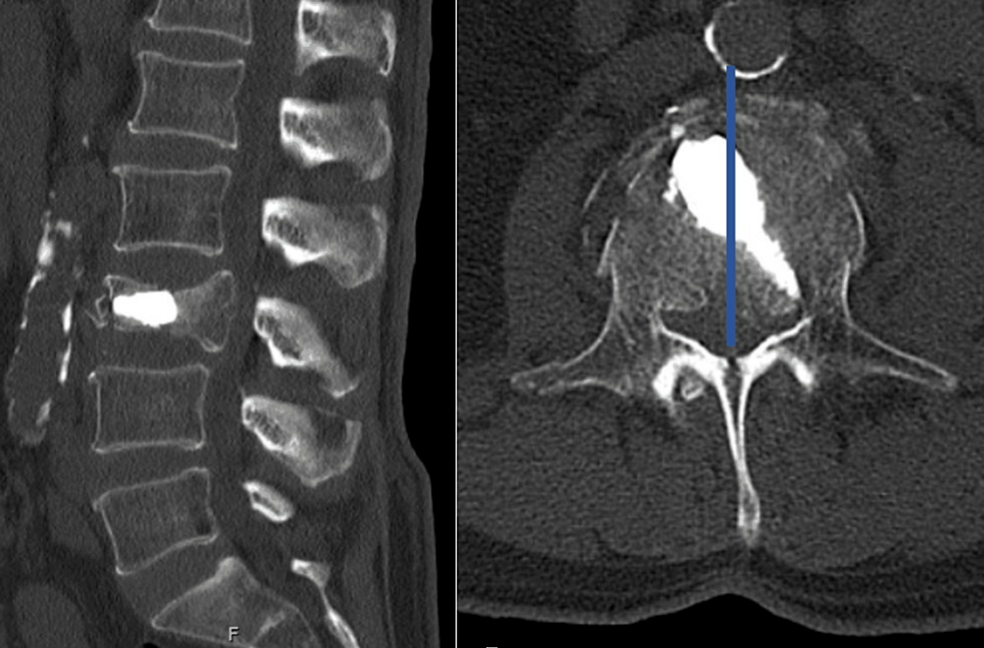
Postoperative CT in sagittal and axial view of combined technique. The line represents the midline
